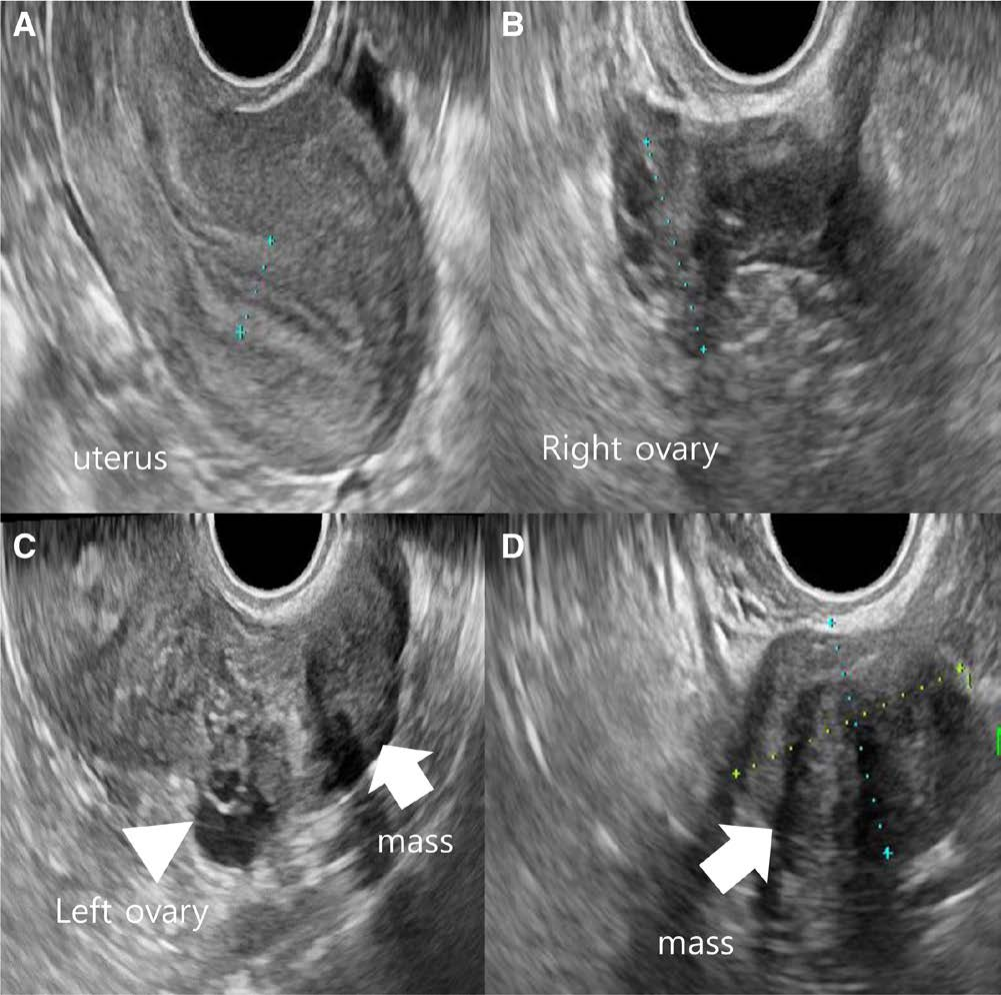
Transvaginal ultrasound findings. (A, B) The uterus and right ovary were normal on transvaginal ultrasound. (C) A well-defined, solid, oval, and hypoechogenic mass (arrow) with stripy shadows measuring 34 × 23 mm was seen next to the normal left ovary (arrow head). (D) There was no vascularity in the mass (arrow) on color Doppler.
